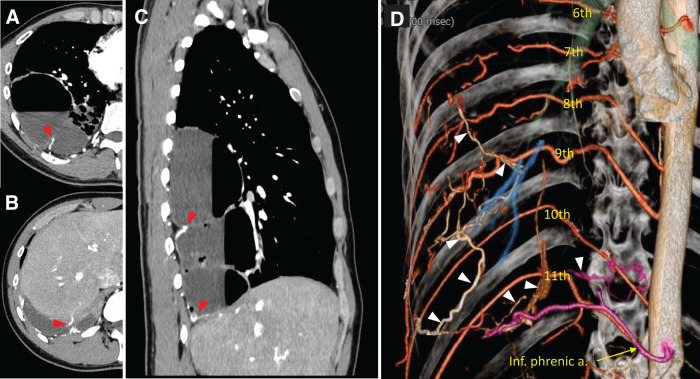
(A–C) Aberrant vascularization flowing into the lung parenchyma from the chest wall, with enhanced blood flow in the cyst walls. (D) 6–11th intercostal arteries and the Inf. phrenic a. Aberrant serpentine feeding arteries (arrow heads) originating from the intercostal arteries and the inferior phrenic artery are detected on CT angiography. Inf. phrenic a., inferior phrenic artery
